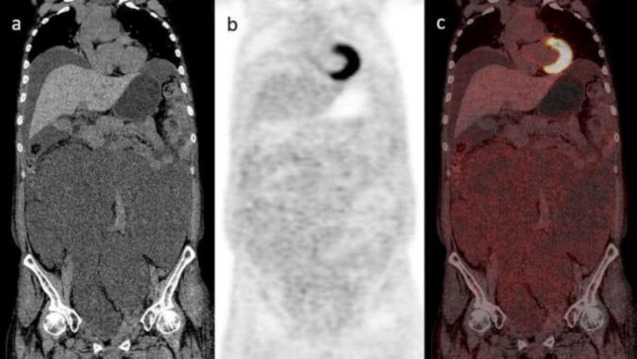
The coronal CT (a), PET (b), and fused (c) images showed multiple low-density masses of different sizes in abdomen pelvic cavity, and mild increased FDG uptake with SUV_max_ of 2.2
